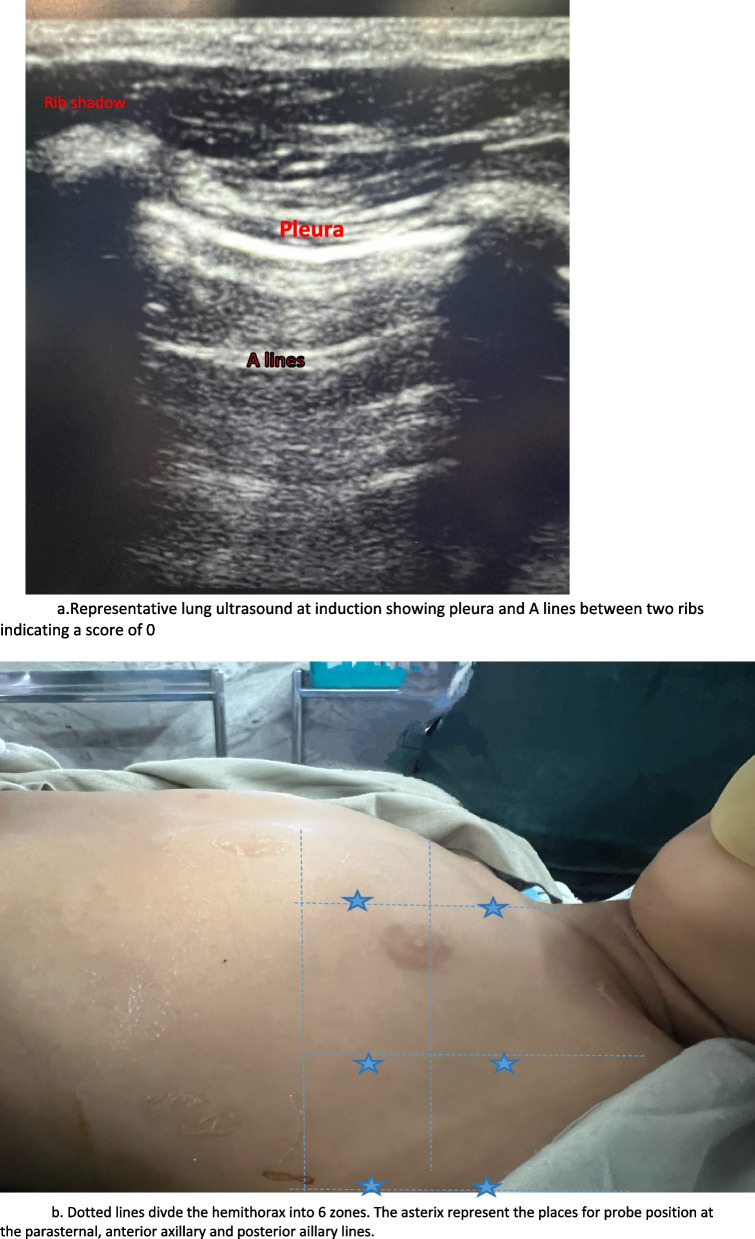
a Lung ultrasound with score 0 1b) Position for probe for lung ultrasound in each hemithorax
